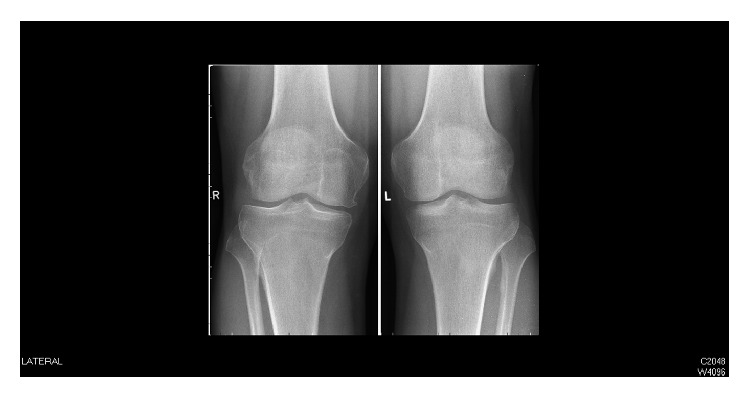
Radiography of the knees shows signs of arthrosis: a bony spur at the medial femoral condyle, femorotibial narrowing of the joint space, and a hydrops.
